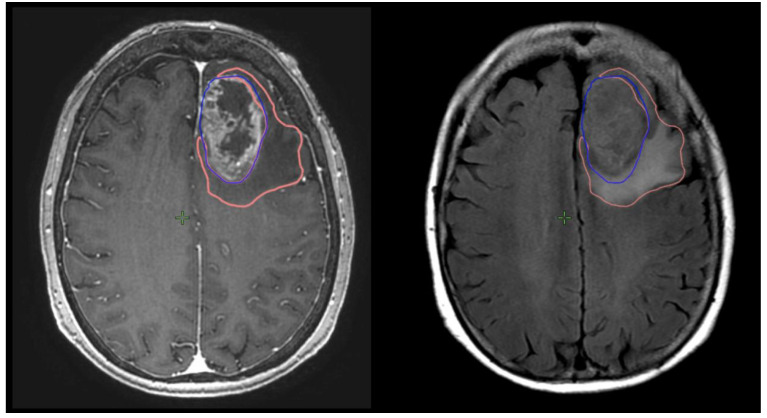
Measurement of T1gd volume (GTVT1gdpre: blue) and T2 volume (GTVT2pre: orange) on respective MRI T1g sequences on right and T2 flair sequence on left.
